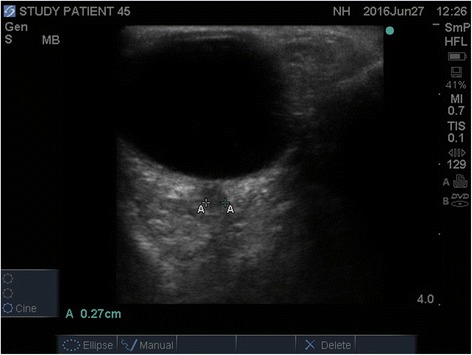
Optic nerve sheath diameter measurement by ultrasound
